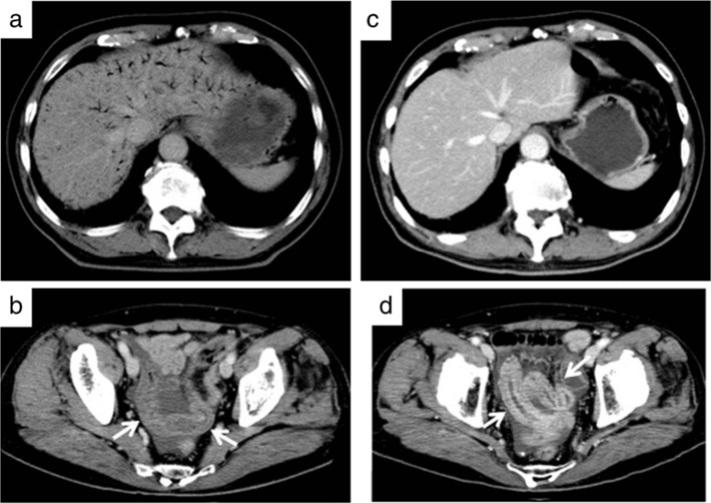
Contrast-enhanced abdominal computed tomography showing hepatic portal venous gas (HPVG) (a) and thickening of the ileum wall (b; *arrow*). Upon readmission to our hospital, the HPVG disappeared (c) but the ileum wall thickening did not improve (d; *arrow*)
